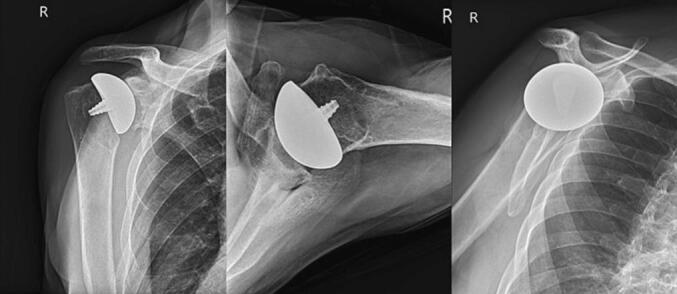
One-year postoperative AP, axillary, and scapular radiographs of the right shoulder in patient I.
